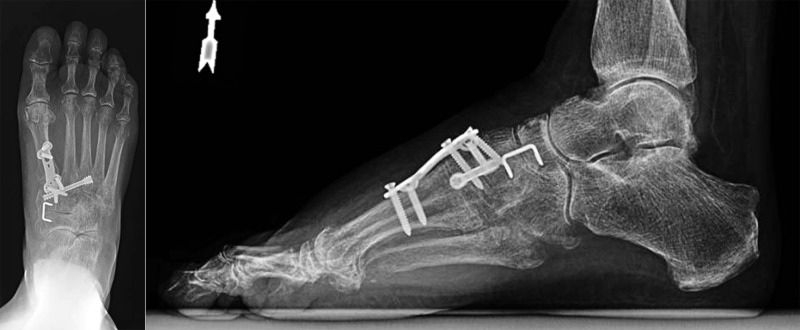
Postoperative lateral radiograph shows fixation with dorsal metatarsophalangeal plate
